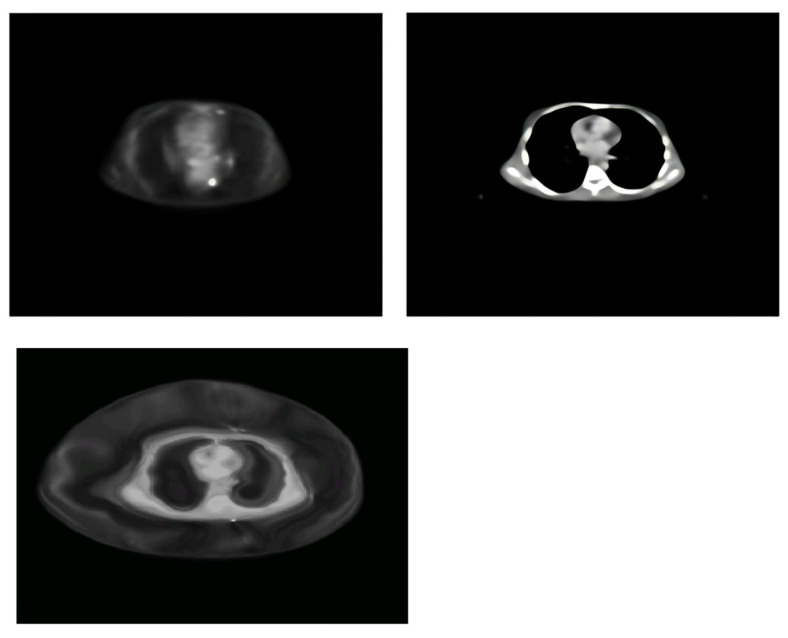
Example of MIRT registration. From LHS: PET images were used as a source, and CT images were used as a reference; the result of registration when using 100 bins is shown. This configuration provided improved alignment of subtle anatomical structures.
